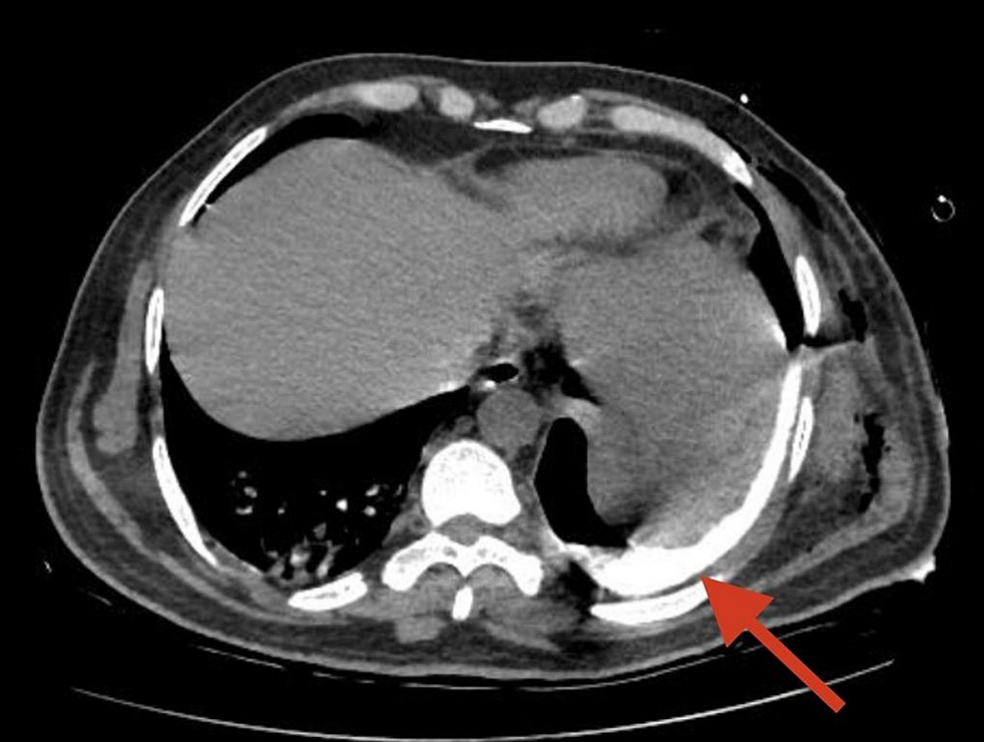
A transverse section of a contrast-enhanced CT showing oral contrast extravasation (red arrow) into the left pleural space, indicating a fistulous opening from the GI tract into the pleural space.
